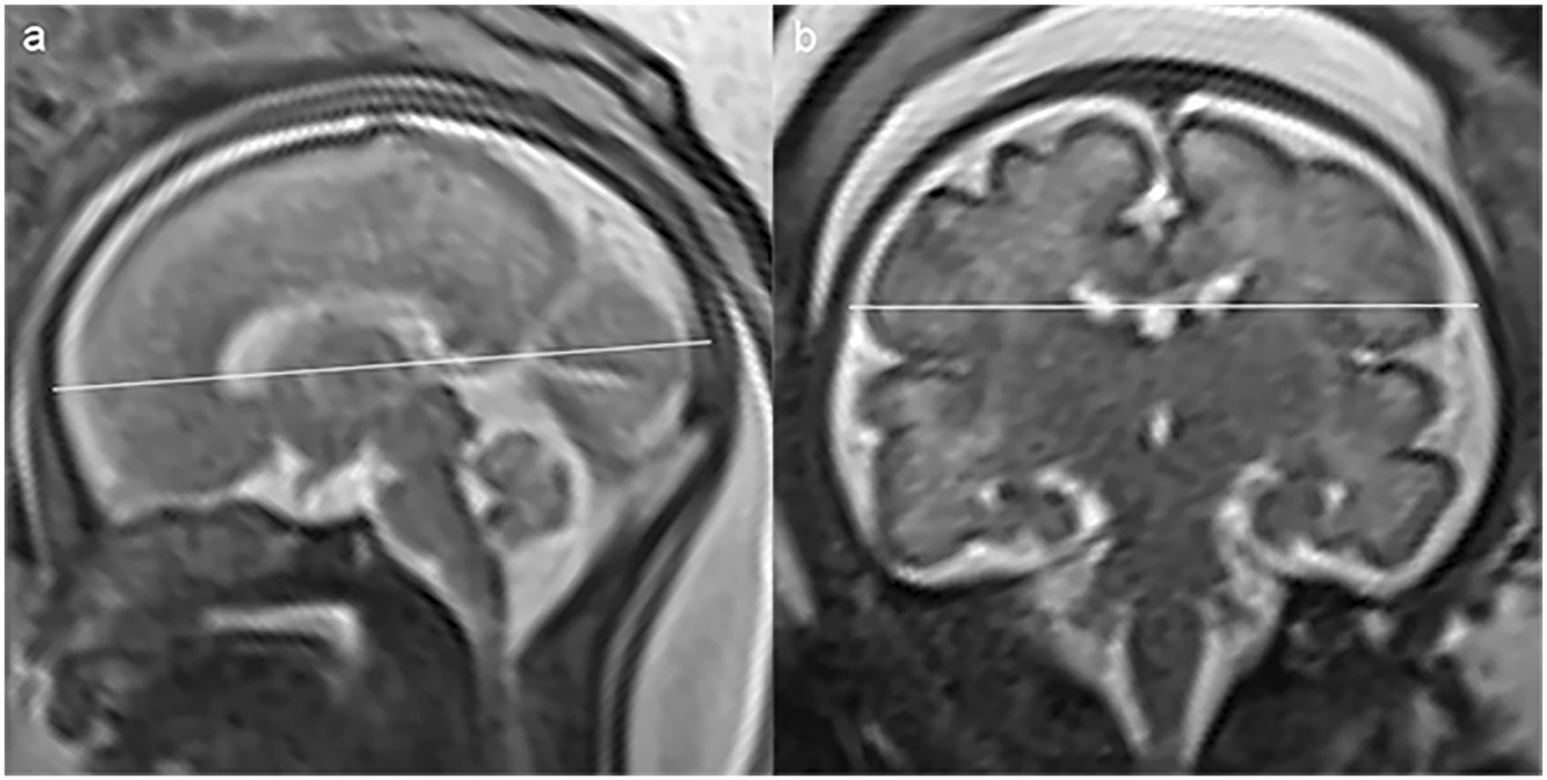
Sagittal and coronal T2-weighted imaging showing the measurements of bFOD (a) and bBPD (b) in our study.
